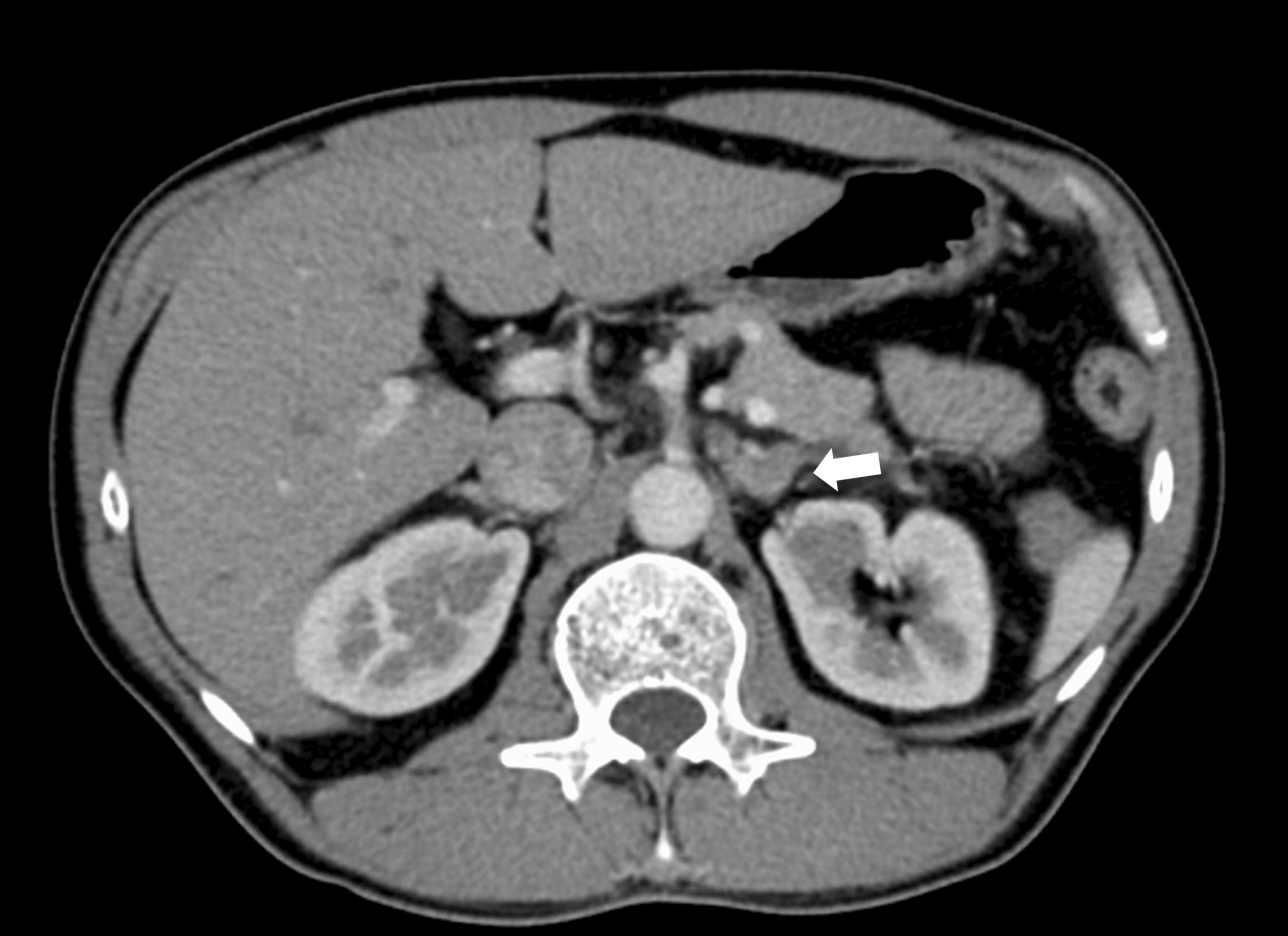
Thoracoabdominal computed tomography with contrast enhancement 16 days before the patient visited our hospital. Imaging revealed mild enlargement of the left adrenal gland and adipose tissue turbidity surrounding the left adrenal gland (arrow).
